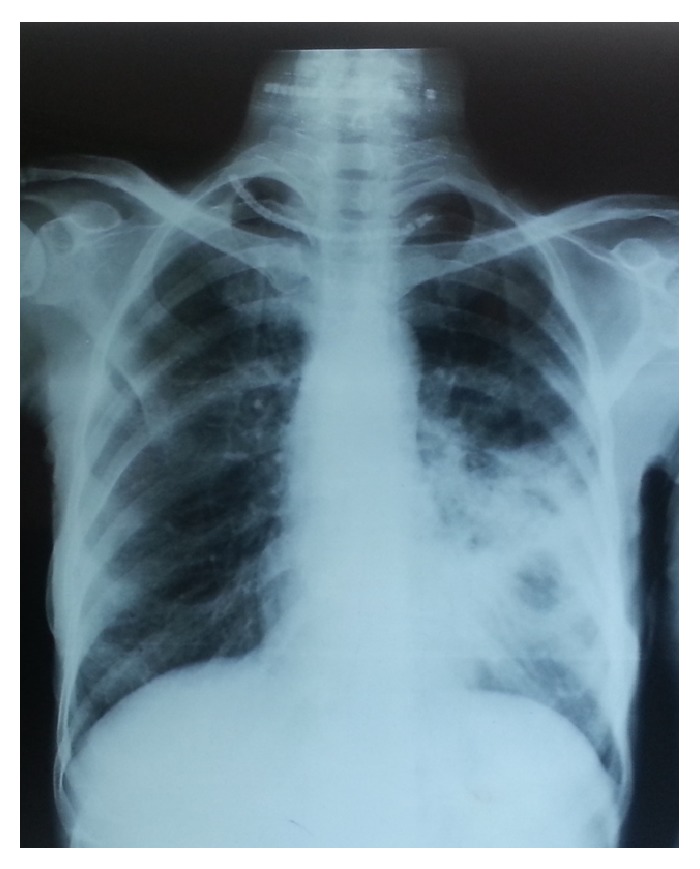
Chest X-ray showing moderately advanced lesion with cavity less than 4 cm in diameter.
